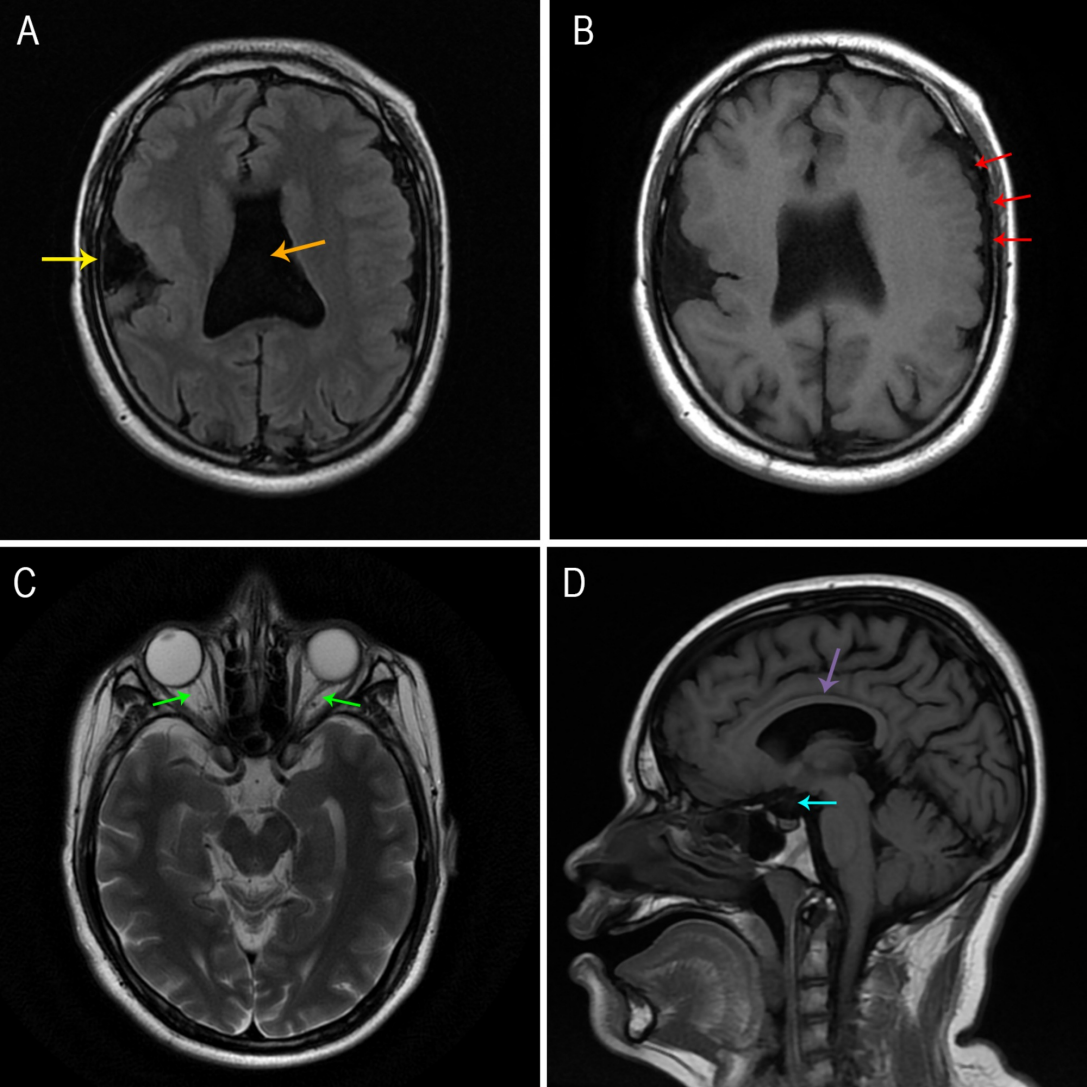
Magnetic resonance imaging Panel A: T2-weighted fluid-attenuated inversion recovery (FLAIR) sequence, axial view. Agenesis of the septum pellucidum, which conditions a “mono-ventricle” appearance (orange arrow) and closed-lip schizencephaly in the right frontal-temporal area, associated with generalized cortical dysplasia of the surrounding parenchyma (yellow arrow). Panel B: T1-weighted FLAIR sequence, axial view. Polymicrogyric appearance and disposition in the left transitional frontal-parietal area (red arrows). Panel C: T2-weighted periodically rotated overlapping parallel lines with enhanced reconstruction (PROPELLER) sequence, axial view. Hypoplasia of both optic nerves (green arrows). Panel D: T1-weighted FLAIR sequence, sagittal view. Hypoplasia of the corpus callosum (purple arrow) and hypoplasia of the pituitary stalk (blue arrow).
